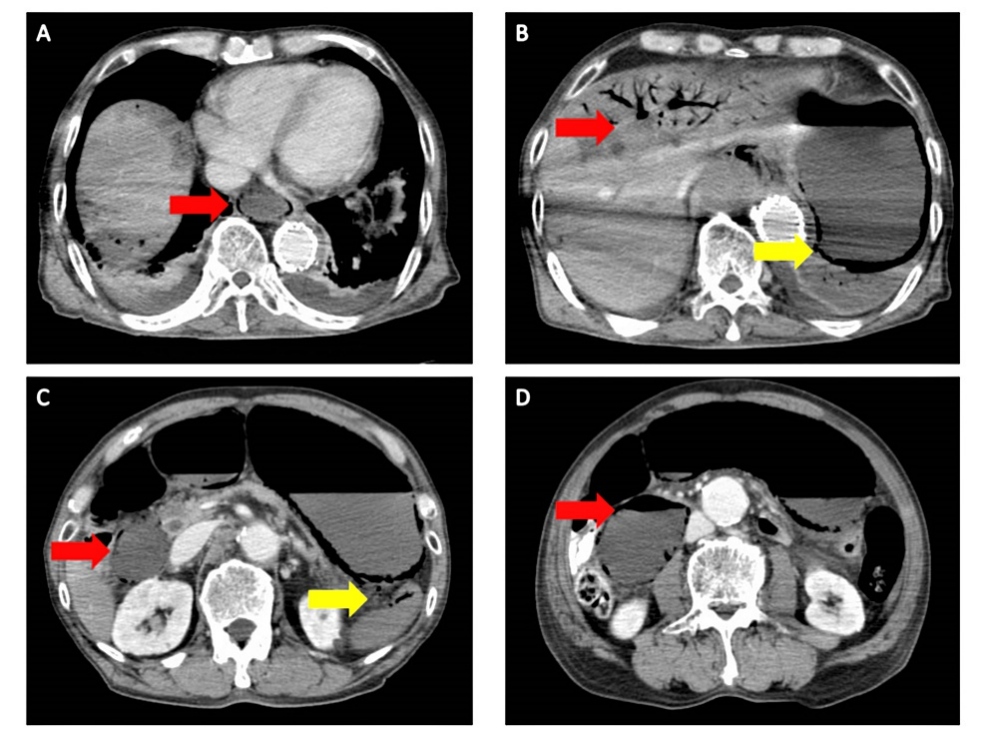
A thoracoabdominal contrast-enhanced CT after TEVAR. There was intestinal emphysema and poor contrast from the lower esophagus to the duodenum (A-D). The lateral hepatic area showed poor contrast and portal vein gas (B). The spleen also showed poor contrast and intravascular emphysema (C). TEVAR: Thoracic endovascular aortic repair.
